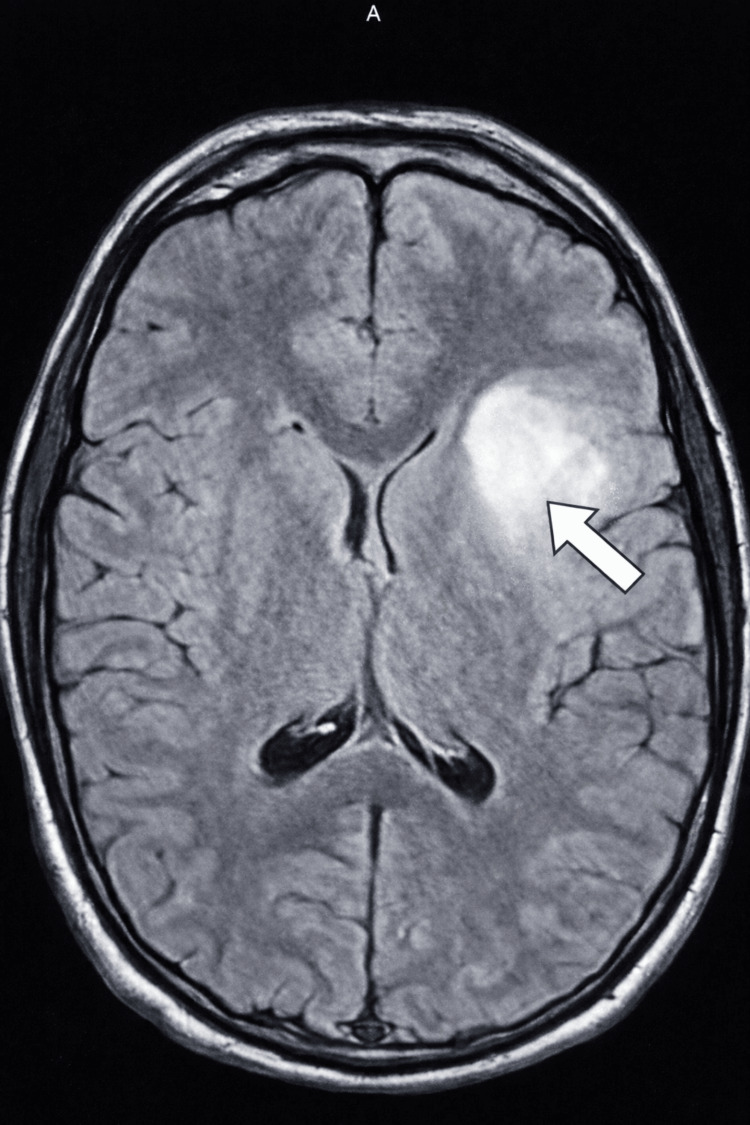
Axial FLAIR MRI of the brain without contrast demonstrates a FLAIR-hyperintense lesion in the left anterior aspect of the external capsule extending into the anterior insular cortex and adjacent frontal white matter (arrow), with imaging features consistent with a low-grade glioma FLAIR: fluid-attenuated inversion recovery; MRI: magnetic resonance imaging
